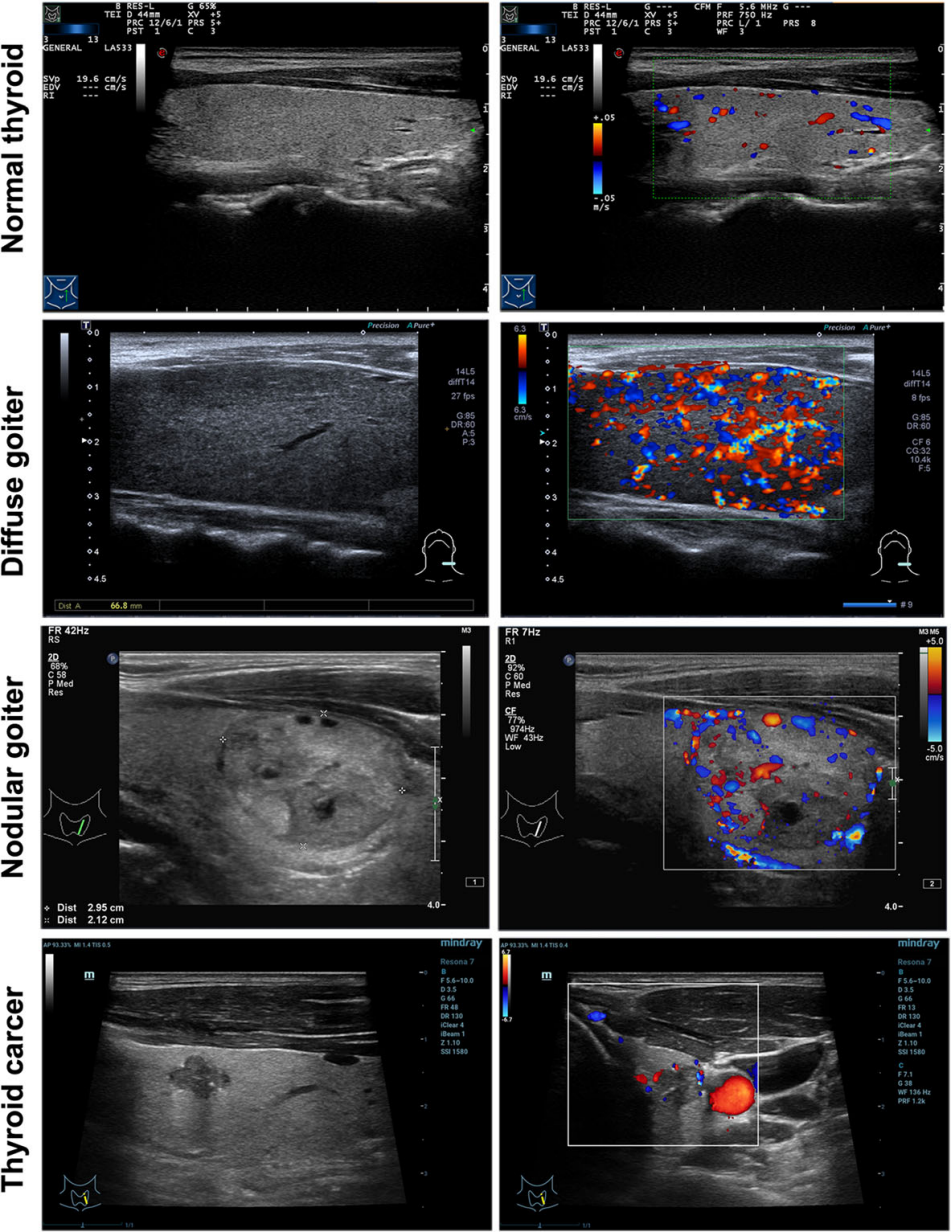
Representative images of nodular goiter and thyroid cancer detected by thyroid ultrasonography
